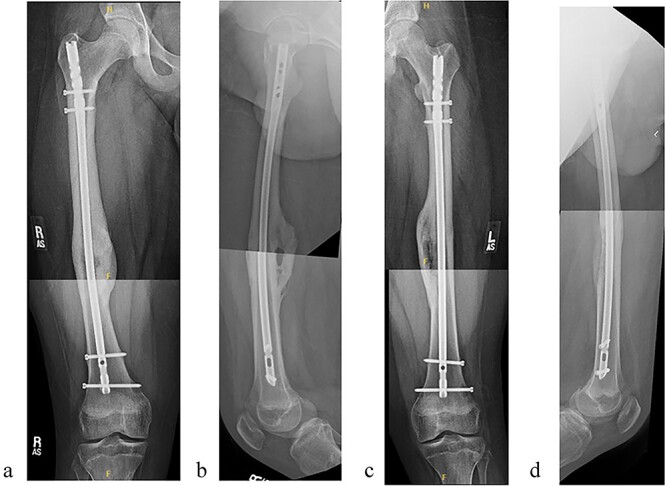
(a) Right femur AP radiograph. (b) Right femur lateral radiograph. (c) Left femur AP radiograph. (d) Left femur lateral radiograph.
